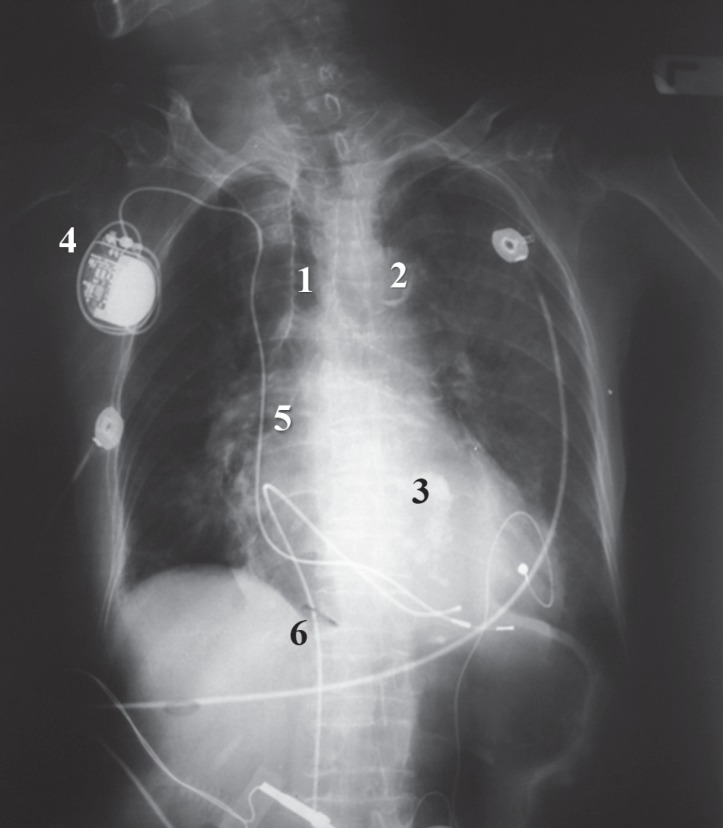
Chest X-ray shows calcification of the trachea (1), aortic arch (2), and mitral annulus (3). It also demonstrates the permanent pacemaker (4), and two leads from the permanent (5) and the temporary (6) pacemakers.
